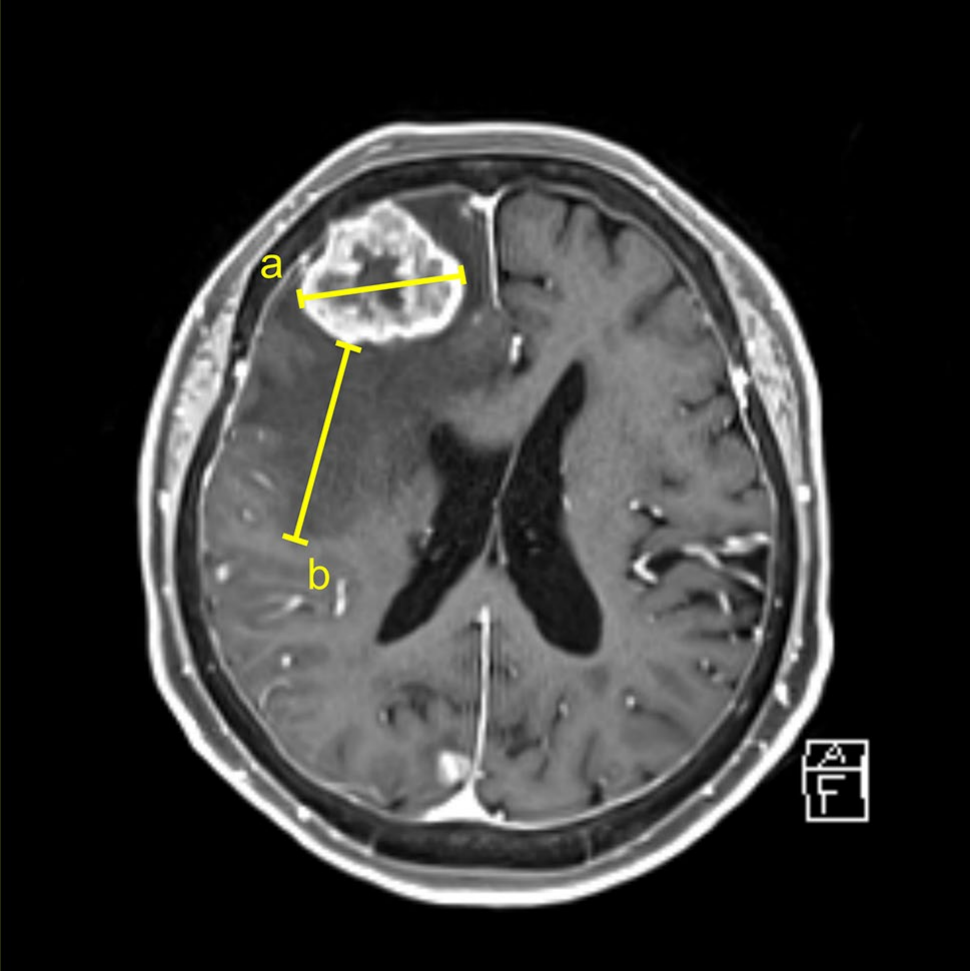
a Maximum tumor diameter was measured on axial contrast-enhanced T1-weighted imaging. b The extent of peritumoral edema was measured on the same slice as the maximum length from the tumor margin to the edge of the edema
